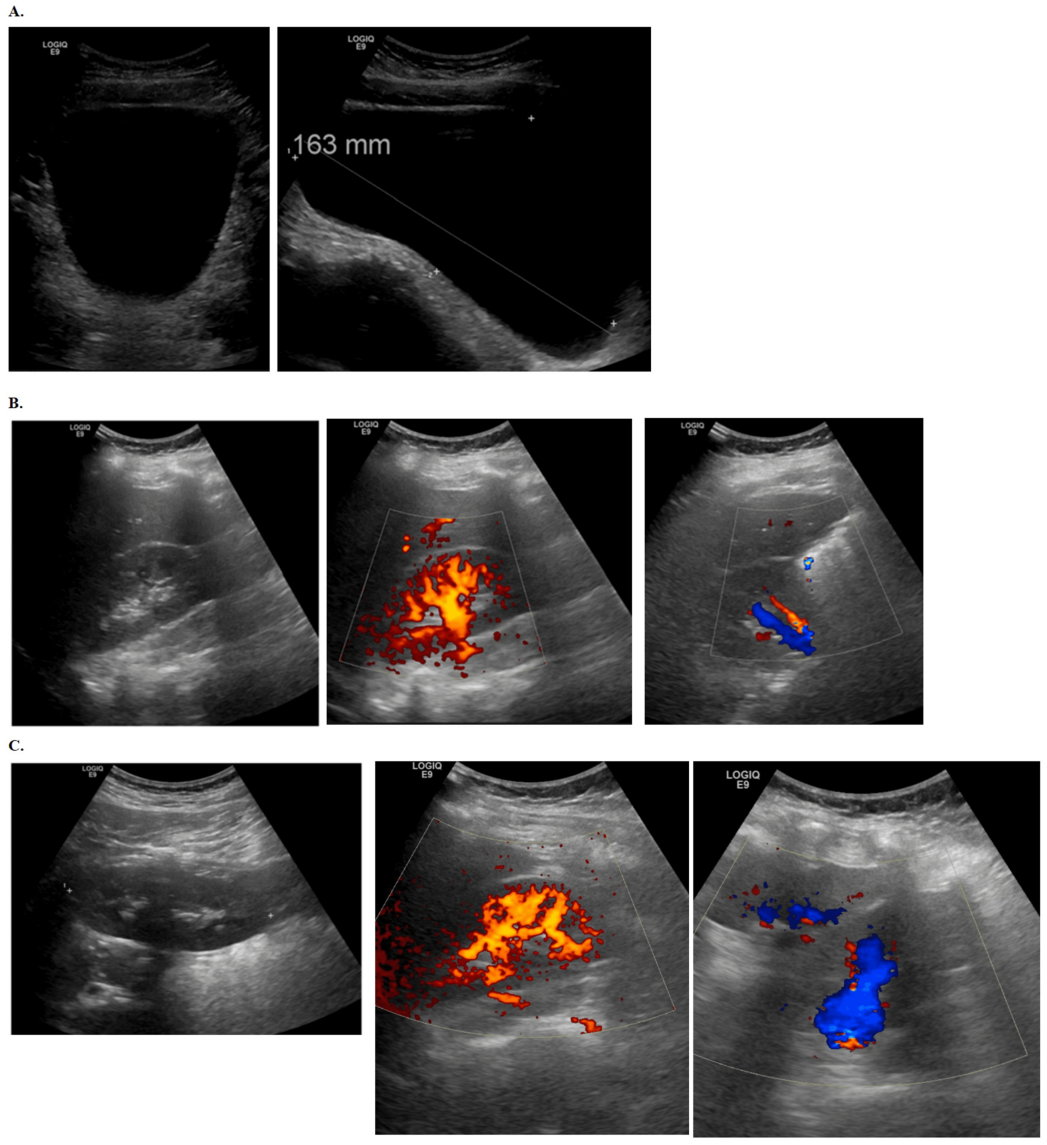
Ultrasound imaging of the bladder and bilateral kidneys A. Sagittal ultrasound view of the bladder showing the bladder wall and bladder lumen. The bladder appears slightly distended due to urine retention, with a measured vertical dimension of 163 mm. B. Transverse ultrasound views of the left kidney with color Doppler, demonstrating blood flow within the renal vessels. The images highlight the vascularity within the kidney parenchyma. C. Transverse ultrasound views of the right kidney with color Doppler. These images show the renal vasculature and confirm normal blood flow, without evidence of obstruction or vascular abnormalities. Note: The kidneys are normal in size, measuring within the range of 9-12 cm bilaterally, with smooth contours and normal parenchymal echogenicity. Corticomedullary differentiation is well maintained, and the renal collecting systems show no dilation or evidence of hydronephrosis. No renal calculi, masses, cysts, or perinephric fluid collections are seen. Color Doppler demonstrates normal renal vascular flow, with no signs of stenosis or thrombosis. No abnormalities indicative of renal parenchymal disease are present.
